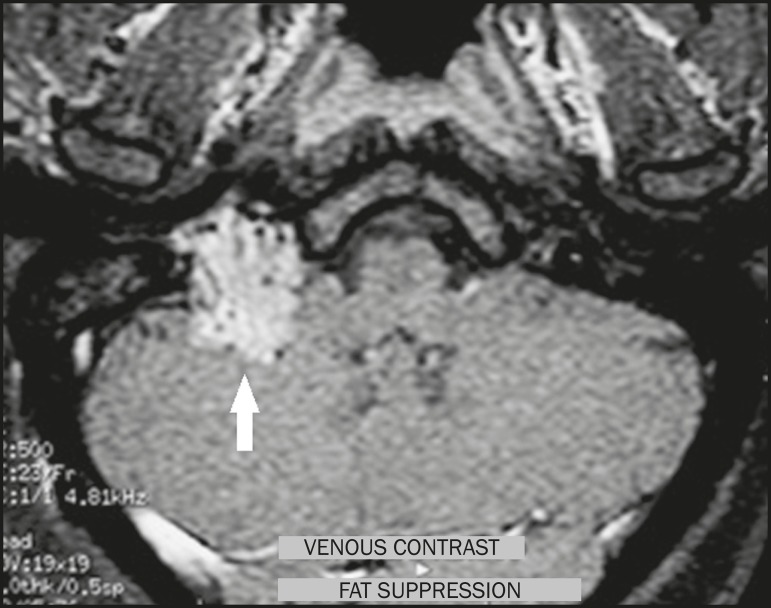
Glomus tumor. Contrast-enhanced axial T1-weighted MRI sequence
